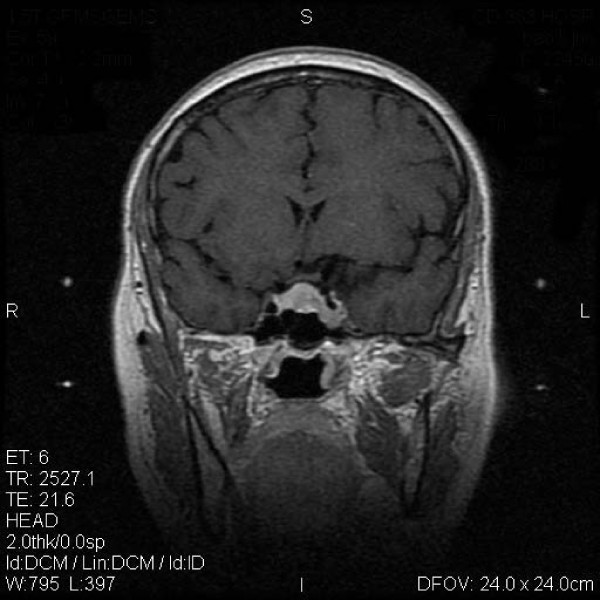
Typical MRI scan changes in GH adenoma. Coronal T1-weighted postcontrast MRI scan at upper left and right, obtained in Patient 3, a 33-year-old man who presented with GH adenomas and acromegaly 7 years before undergoing MASEP GKRS. (Figure 5) An enhancing mass lesion is seen in the sella turcia with extension into the left cavernous sinus. Patient 3's serum growth hormone level was 497.3 ng/ml initially. He was treated with transsphenoidal surgery, and the tumor relapsed shortly with the serum growth hormone level reduced to 130.2 ng/ml. The patient was treated with MASEP GKRS, and MRI was performed for treatment planning. 20 Gy defined to the 50% isodose line is used to cover the full extent of the pituitary tumor in the first radiosurgery, and 28 Gy defined to the 50% isodose line is used to cover the pituitary tumor in the second time one year later.
